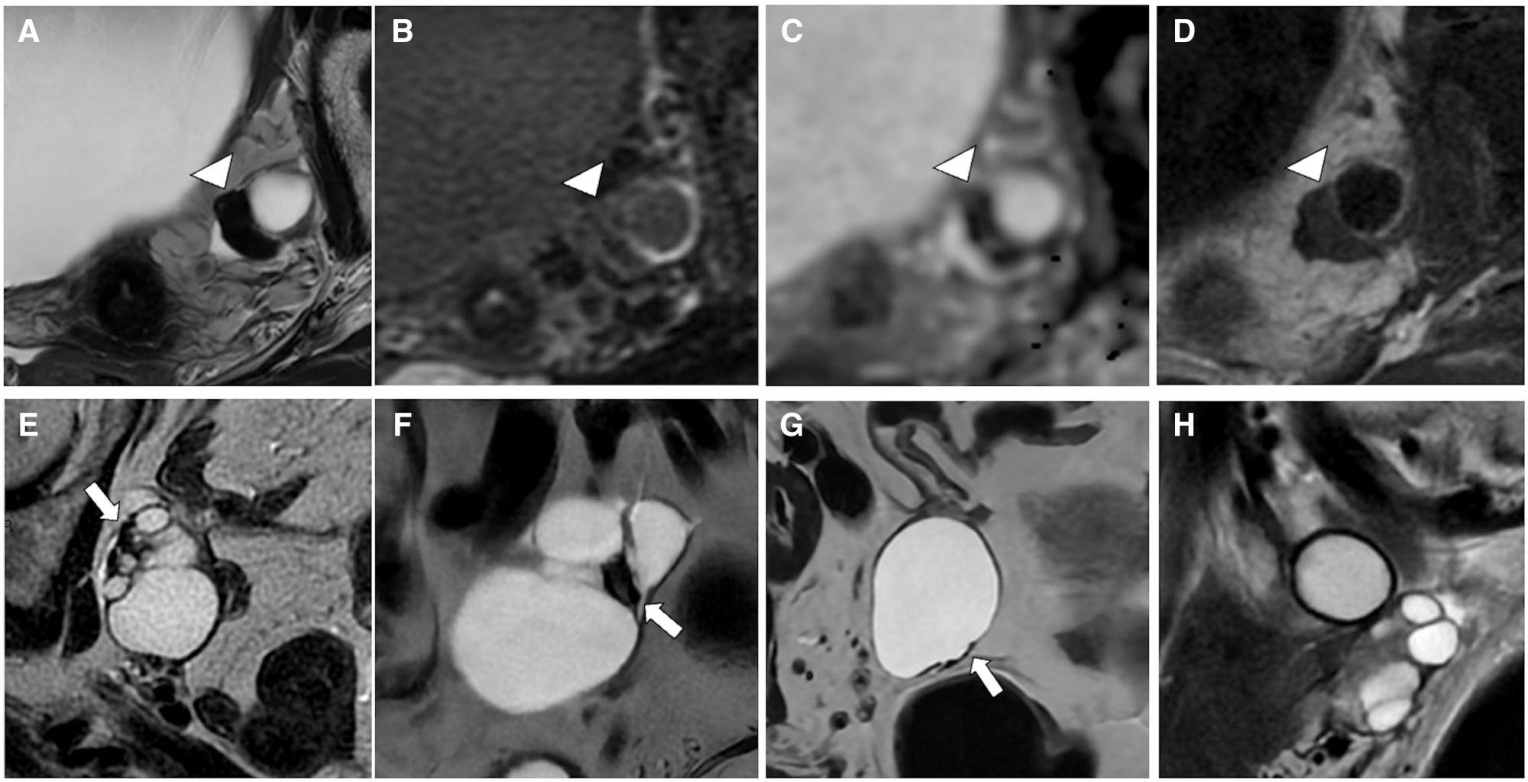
Cystadenofibroma. Pelvic MRI showing a multilocular cystic lesion with a nodular solid component (arrowhead) (A-D). The solid component has low SI on T2-WI (A) and no impeded diffusion (B, C). On post-contrast T1-WI (D), mild and late enhancement is seen. Three morphological patterns of cystadenofibroma have been described. Type I or “black sponge” (E, F): multilocular cystic lesion with fibrotic nodular solid component (very low SI on T2-WI) along the septa (arrow in E) or protruding into the loculi (F). Type II or “carpet-like” (G): unilocular cystic lesion with a plaque-like thickening of the cystic wall (arrow). Type III or “purely cystic” (H): no solid component can be noted in this pattern. Abbreviations: SI = signal intensity; WI = weighted imaging.
